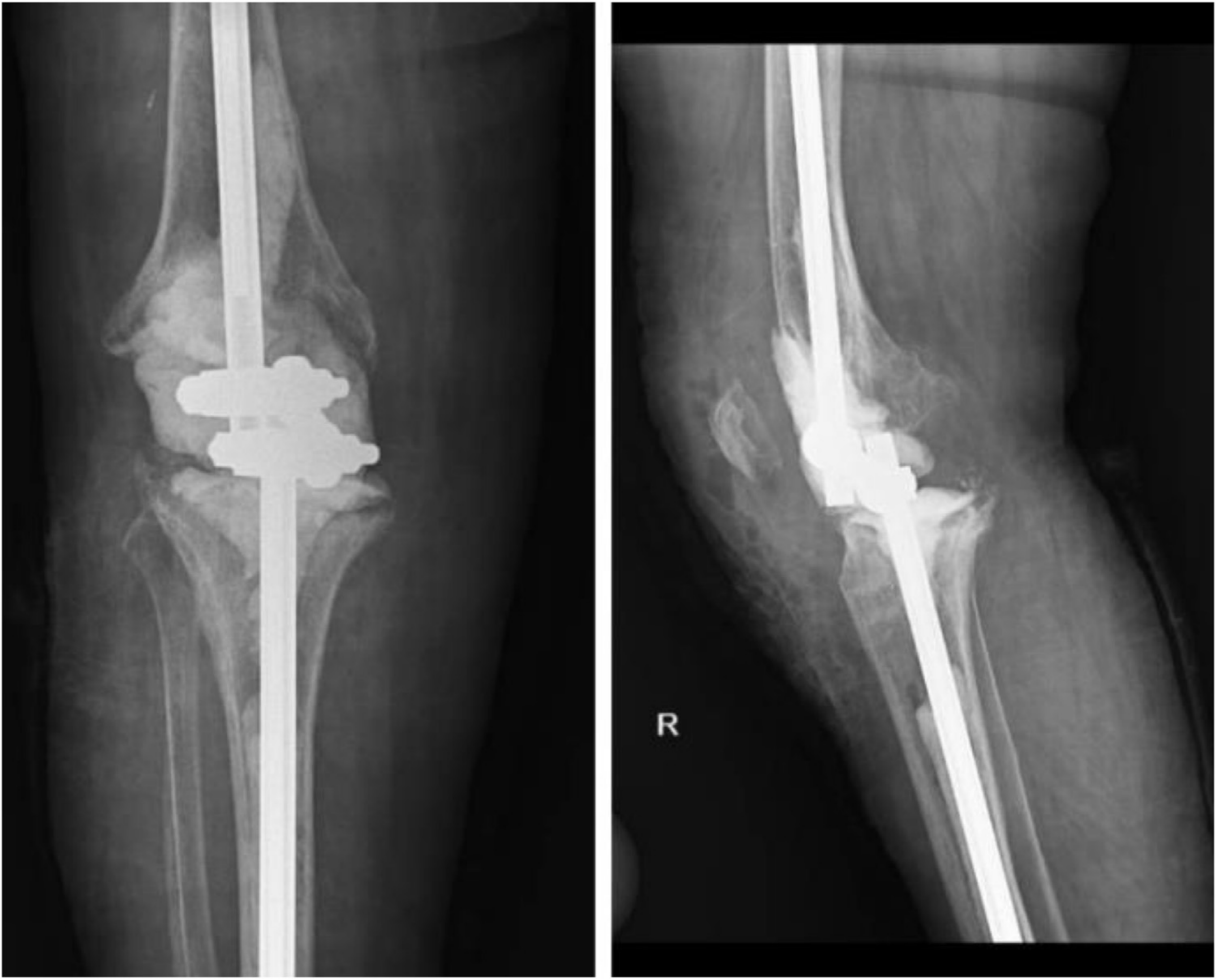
Anterioposterior and lateral postoperative knee radiograph.
